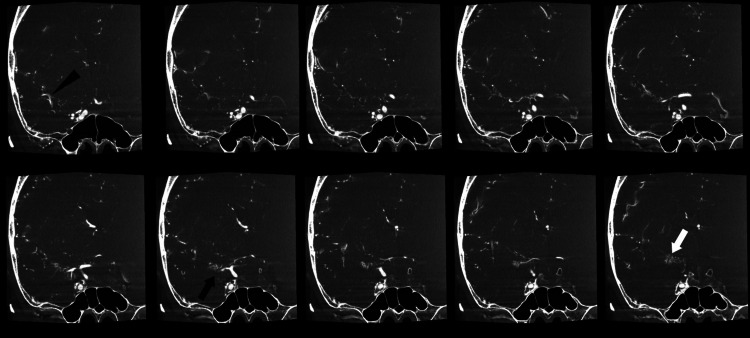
Cone beam CT performed three months after hospital discharge. Black arrow: plexiform arterial network. Black arrowhead: cortical branch of the middle cerebral artery. White arrow: lenticulostriate arteries arose from the plexiform arterial network.
